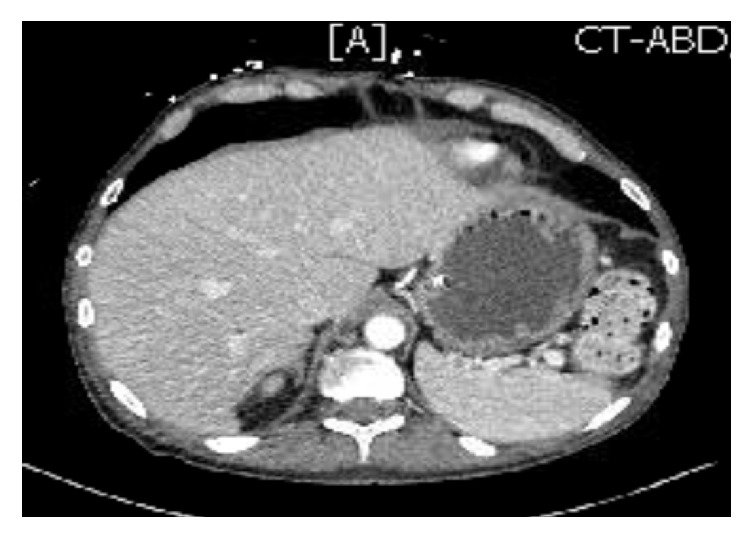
CT scan of the abdomen on the 5th day of admission showing resolution of air in the stomach wall and portal venous gas.
